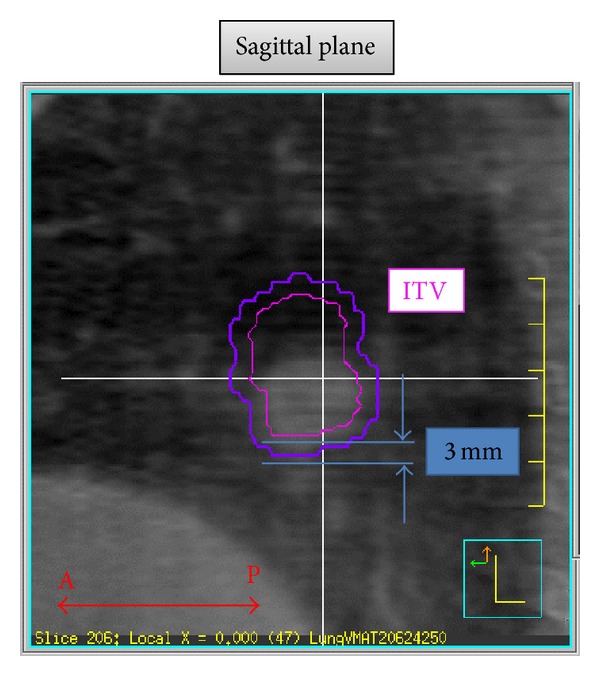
Example of the detection of sagittal displacement between the ITV and the actual tumor location, for which the ITV contoured on the planning CT was superimposed onto the in-treatment 4D CBCT image.
